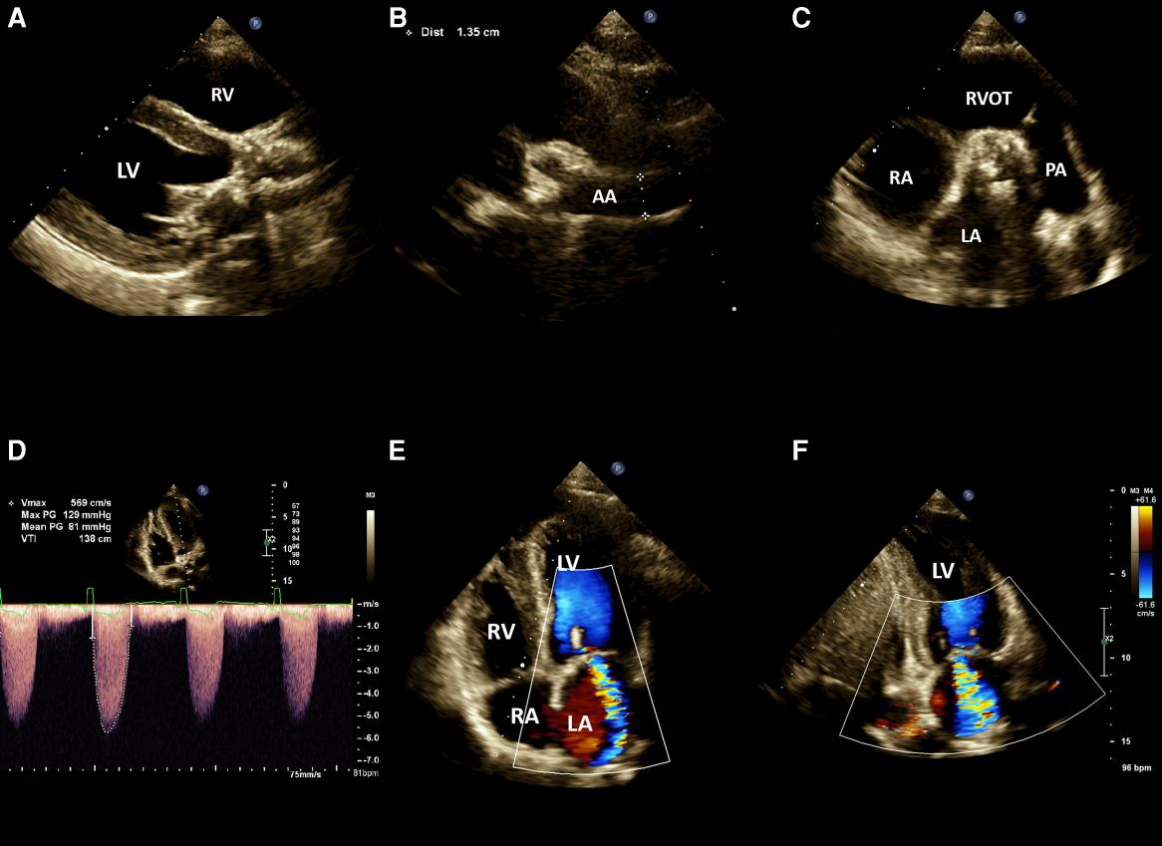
Trans-thoracic echocardiography demonstrated a normal left ventricular size with a moderate symmetrical increase in left ventricular wall thickness, suggestive of concentric left ventricular hypertrophy, as observed in the parasternal long-axis view (*A*). The examination also revealed a small aortic root and ascending aorta (∼14 mm) and a small aortic valve orifice with significant calcification and a marked increase in trans-valvular velocity (5.7 m/s), indicative of both valvular and supra-valvular aortic stenosis (*B*–*D*). In the apical two- and four-chamber views, there was severe trans-valvular mitral regurgitation (*E* and *F*). AA, ascending aorta; LA, left atrium; LV, left ventricle; PA, pulmonary artery; RA, right atrium; RV, right ventricle; RVOT, right ventricular outflow tract.
